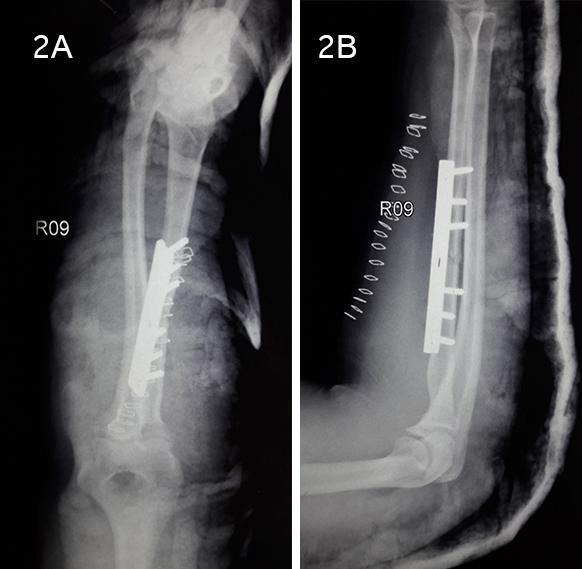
A, B. Immediate postoperative plain radiographs after radial head reduction and fixation of shaft fracture (A) Anteroposterior view (B) Lateral view.
